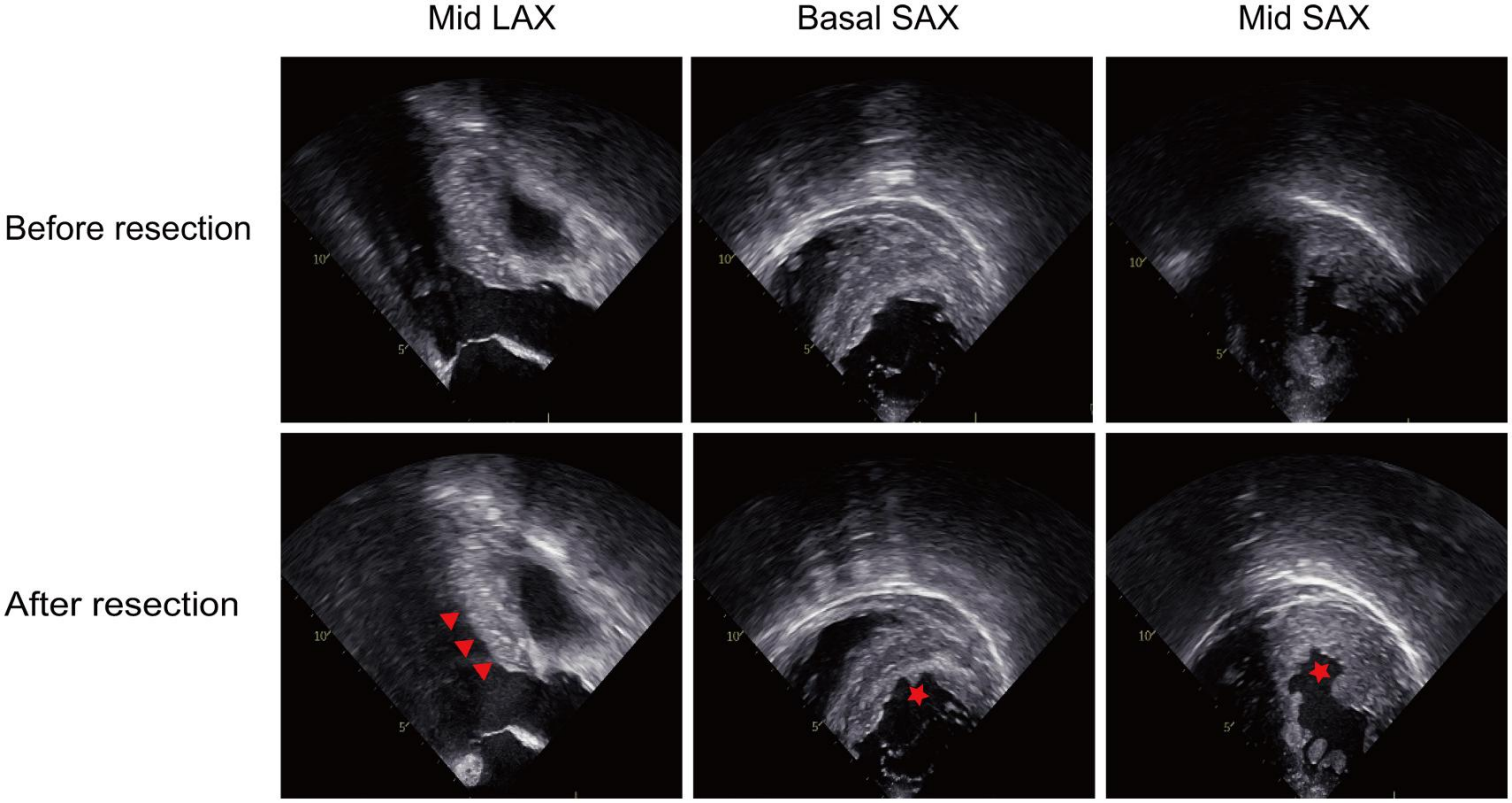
Intraoperative transoesophageal echocardiography images before and after resection. The scar created by transapical beating-heart septal myectomy is marked by red arrowheads in LAX or red pentagram in SAX. LAX: long-axis view; SAX: short-axis view.
